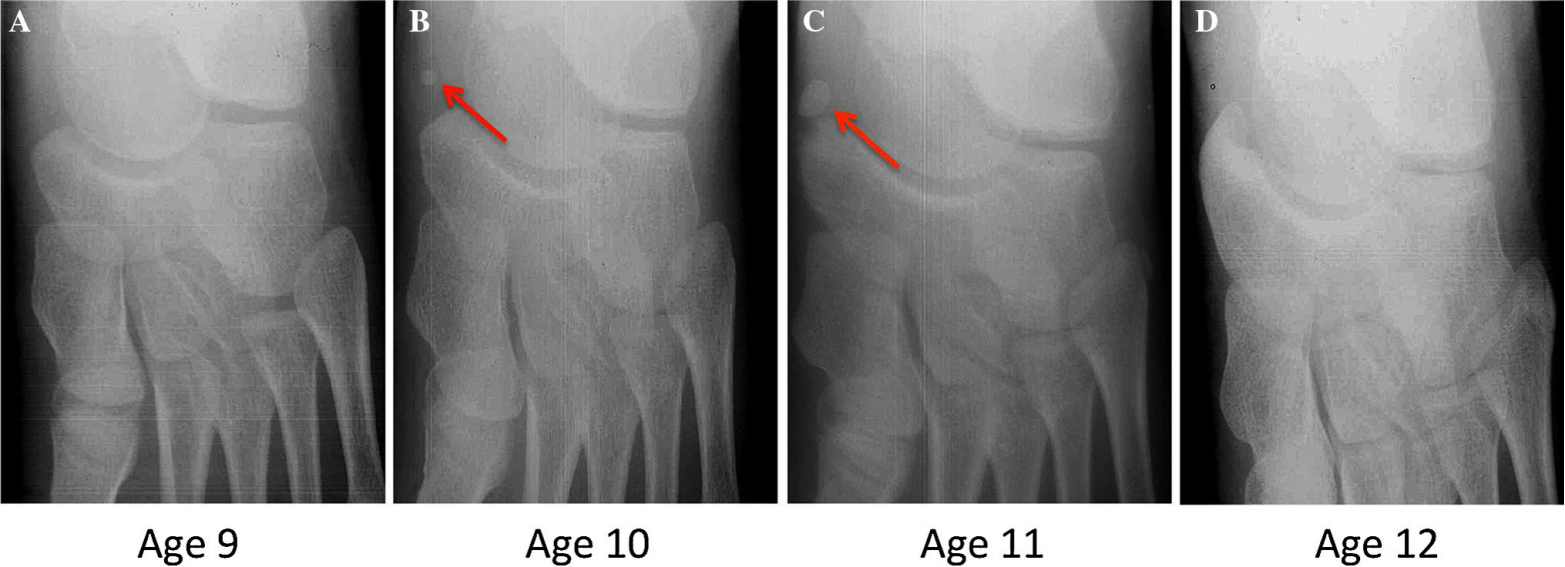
Serial radiographs of an asymptomatic male patient showing no radiologic evidence of accessory navicular (a), the initial appearance (b, *red arrow*), accessory ossicle growth with continued discontinuity (c, *red arrow*), and osseous fusion (d)
